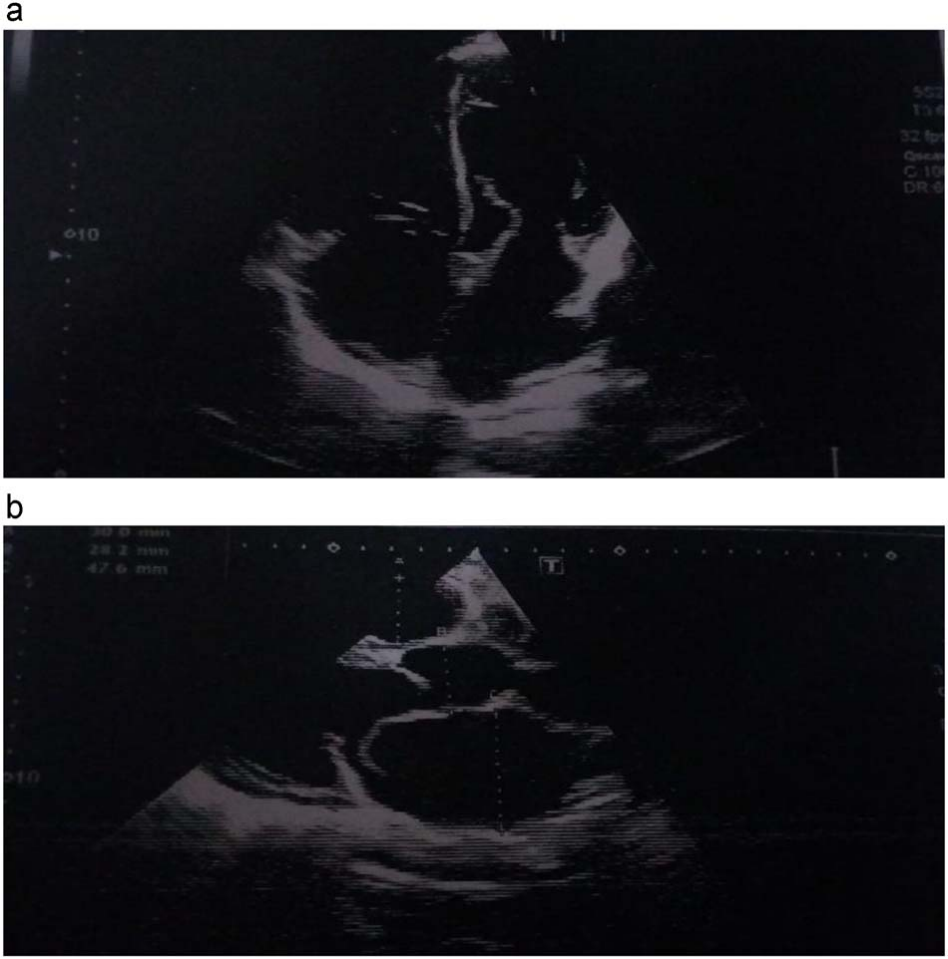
(a, b) Echocardiographic picture showing the avulsed papillary muscle.
